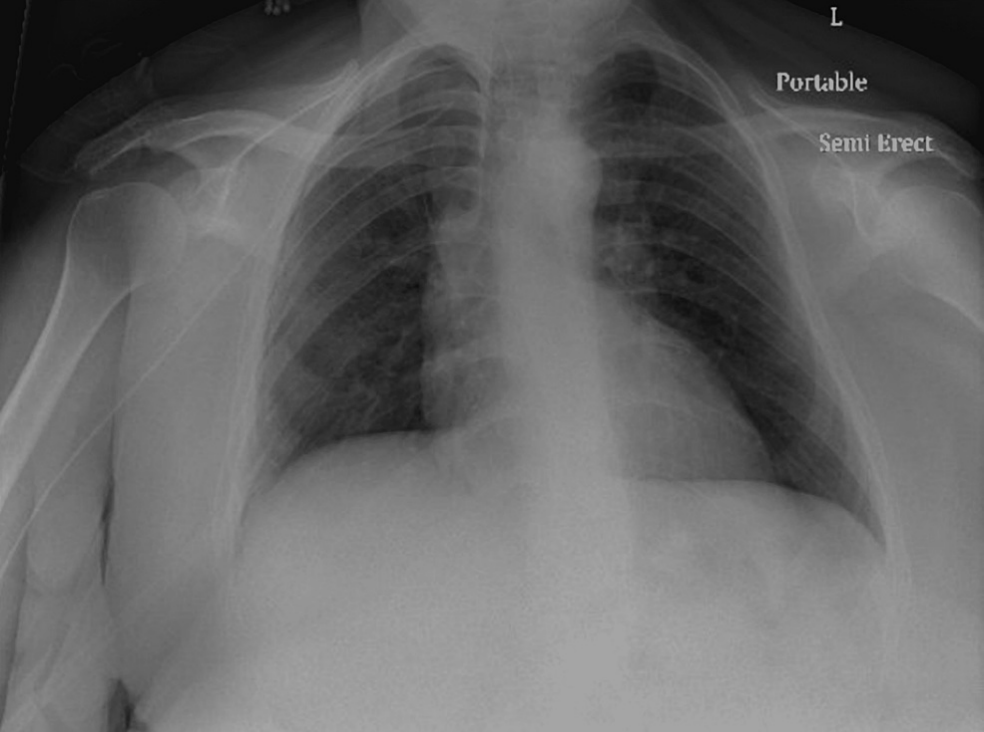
Chest X-ray prior to discharge revealing resolution of the lung opacities bilaterally.
